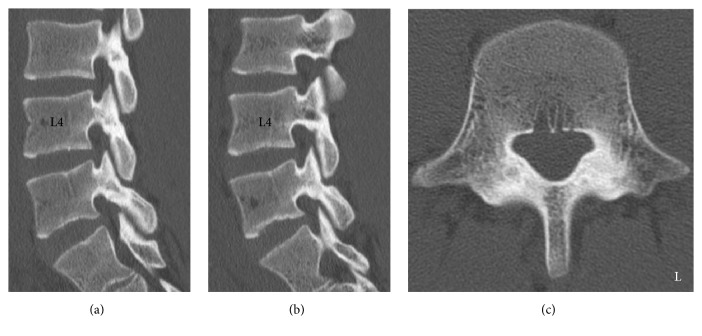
A 1-year follow-up reconstructed computed tomography (CT) scan of the lumbar spine after initial conservative treatment. (a) Right parasagittal reconstructed CT image. (b) Left parasagittal reconstructed CT image. (c) Axial CT image at L5 level. The radiological findings indicated that the bilateral spondylolysis of the L5 had united (a, b, c).
